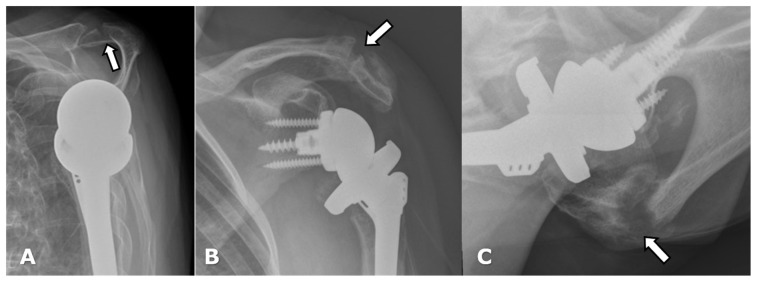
(A) Scapular Y radiograph in a patient with an RTSA shows an acute acromial fracture (arrow). (B) Frontal and (C) axillary radiographs in a different patient demostrate a healing acromial fracture (arrow) following RTSA.
